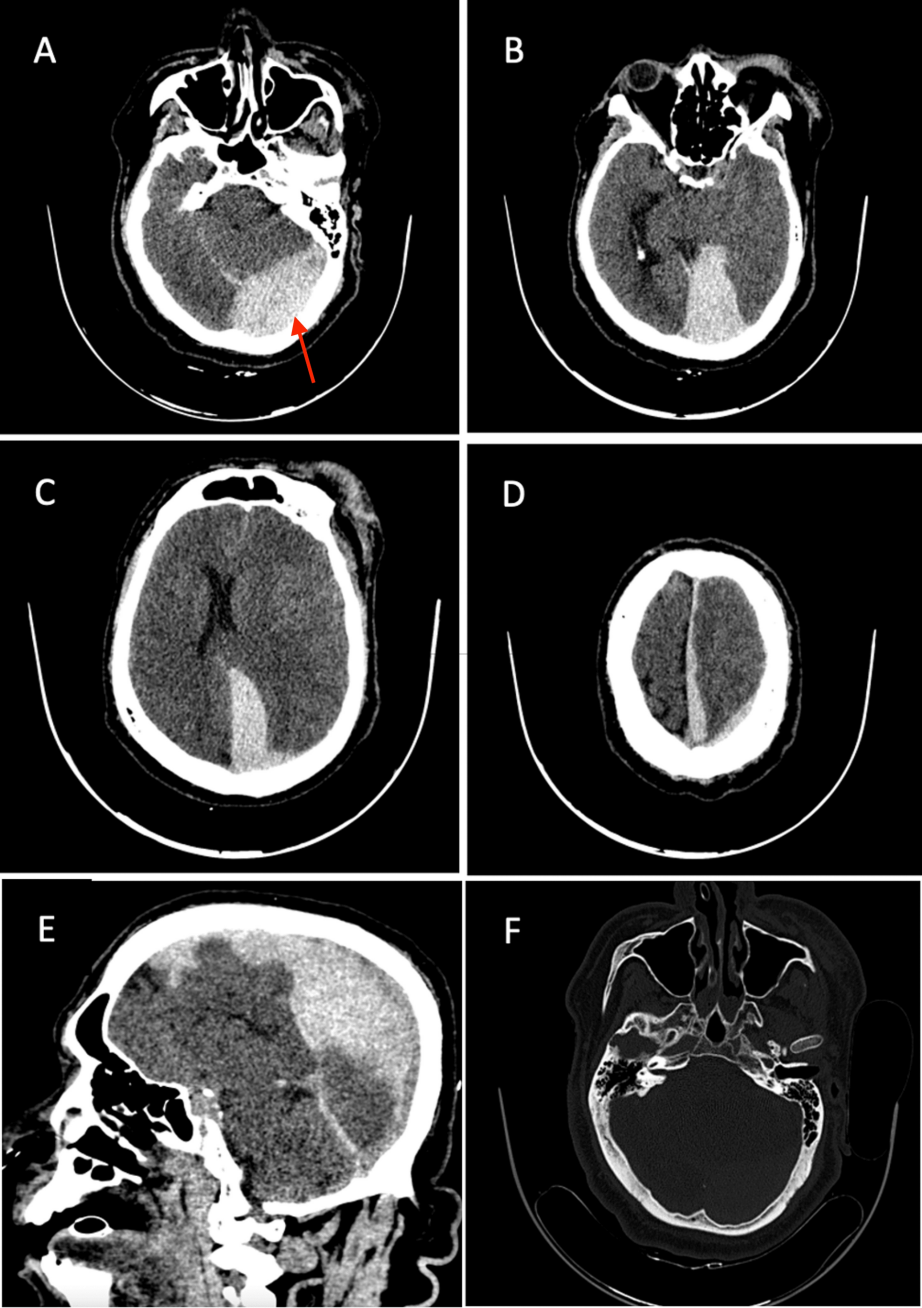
Preoperative plain head CT scan A-D) Axial view showing the acute subdural hematoma in the tentorial and interhemispheric region. Cerebral edema present on the left frontotemporoparietal area with midline shift of 14mm. E) Sagittal view. F) Bone window, level of torcula with no skull fracture. The red arrow indicates the location of the hematoma near the left transverse sinus, suggestive of venous sinus injury, which was confirmed intraoperatively.
